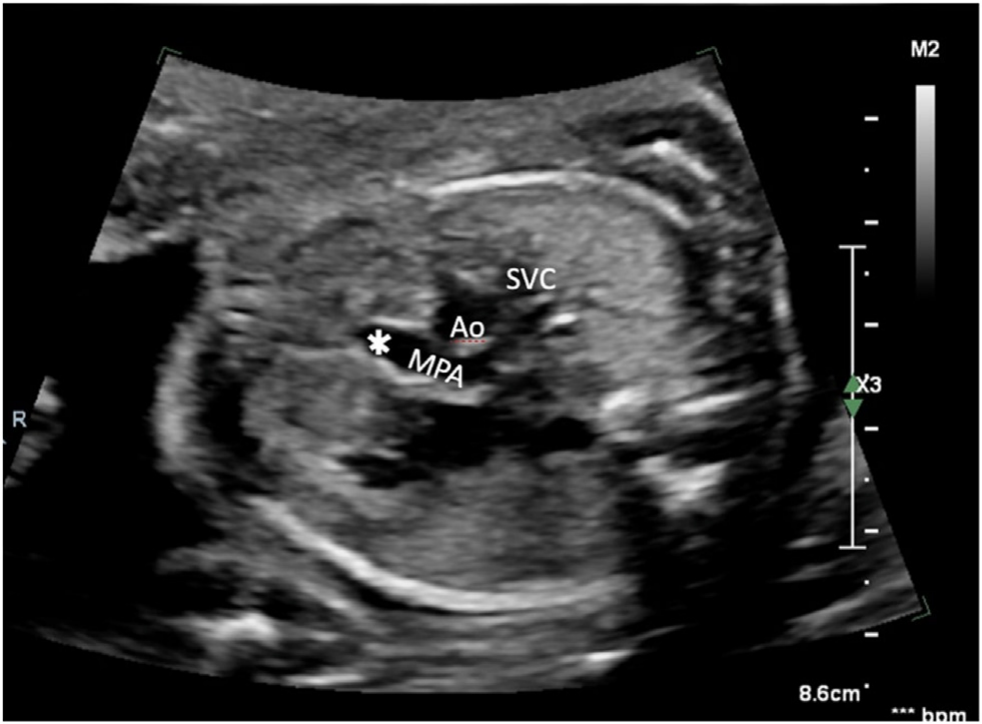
Transabdominal two-dimensional fetal echocardiogram, outflow tract view, demonstrates a normal-sized MPA and no evidence of PV leaflets (*asterisk*). *Ao*, Aorta; *SVC*, superior vena cava.
